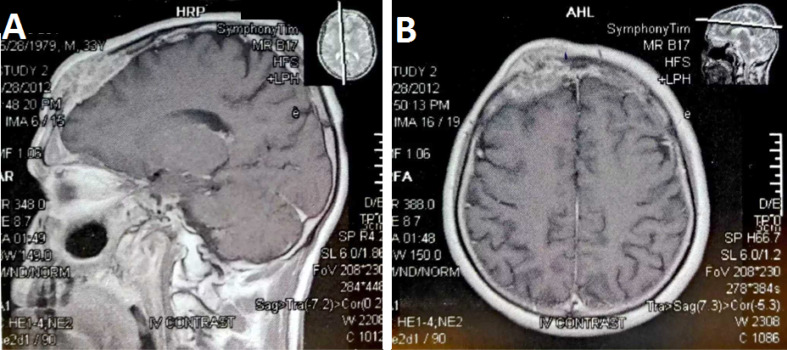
The patient was followed-up clinically and serial CT scans or MRI periodically until seven years. Follow-up imaging showed a gradual regression of paranasal sinuses tumor, and he is completely clinical disease-free for approximately seven years.
